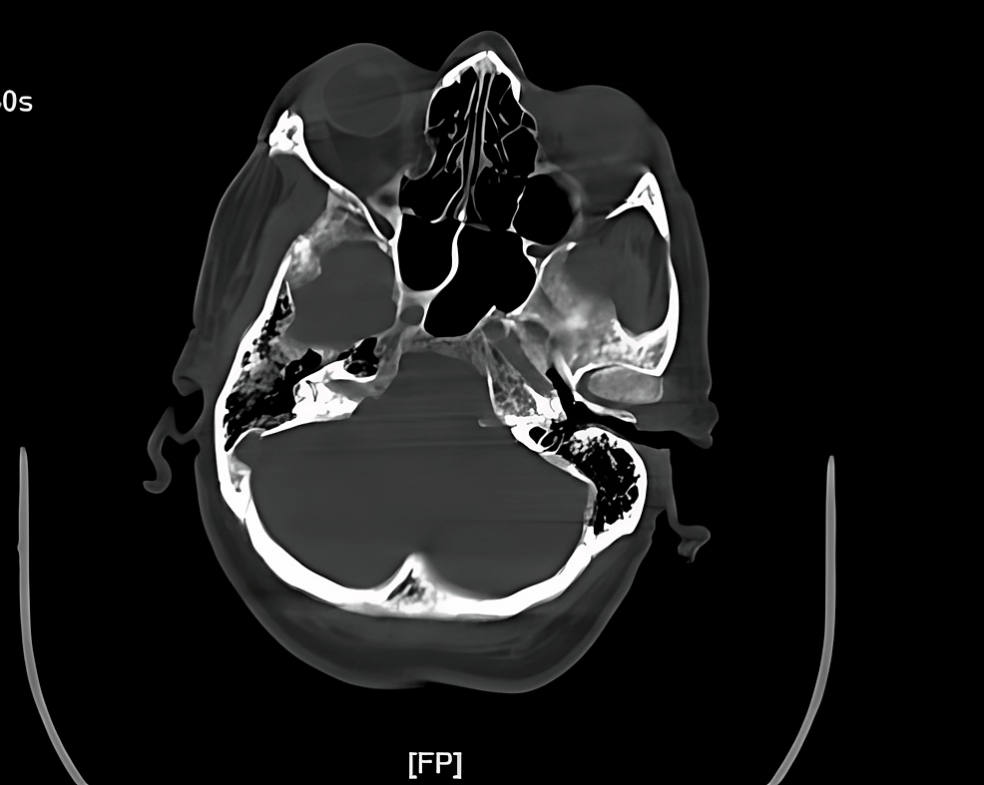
Axial CT without contrast scan of the sinuses taken in 2020.
